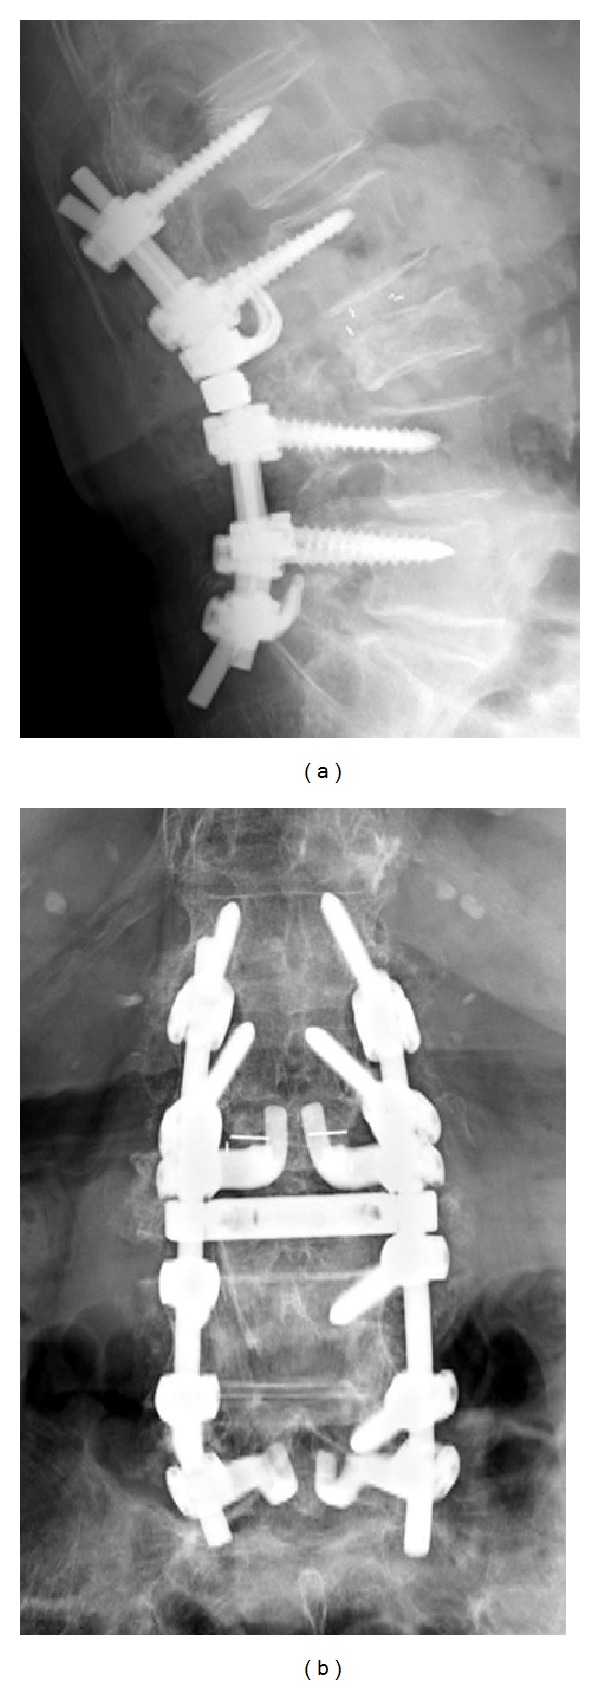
Posterior decompression and fusion surgery was performed on the 85-year-old woman with an L3 vertebral body fracture. Postoperative anteroposterior (a) and lateral (b) radiographs show appropriate positioning of intervertebral cages and pedicle screws.
